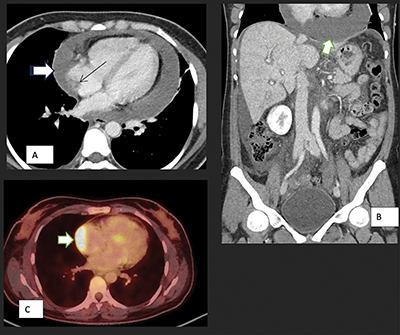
Axial (A) and coronal (B) CT scan images show pericardial effusion (white arrow) and enhancing pericardium, evidence of right heart failure with IVC enlargement and periportal oedema, features of early cardiac tamponade. The thin arrow points to the soft tissue mass in the atrium. Axial PET-CT (C) shows pathological metabolic activity in the known mass in the right atrium (arrow) extending along the pericardium with intensely increased FDG uptake.
